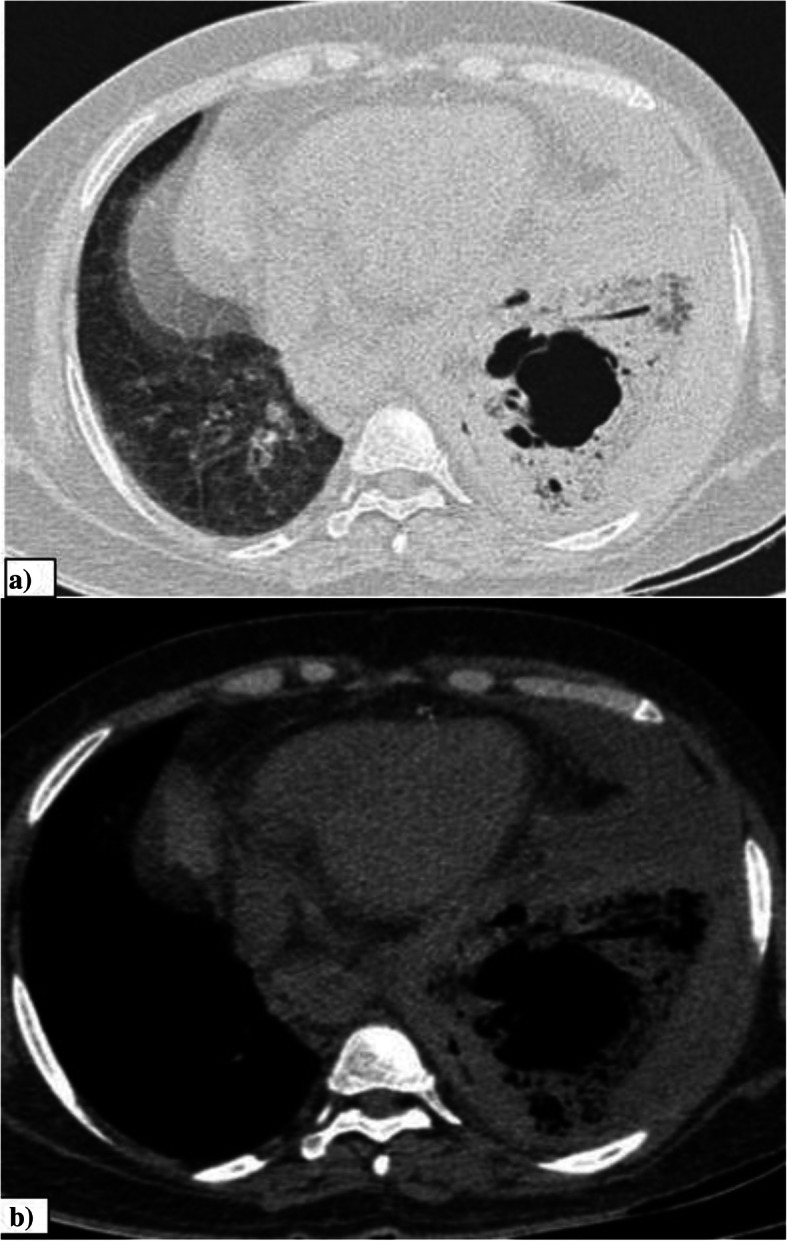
Necrotizing pneumonia. a, b Axial CT thorax (lung window and mediastinal window) of a 51-year-old COVID pneumonia patient areas of cavitation in the background of consolidation in the left lower lobe. Left pleural effusion also noted
